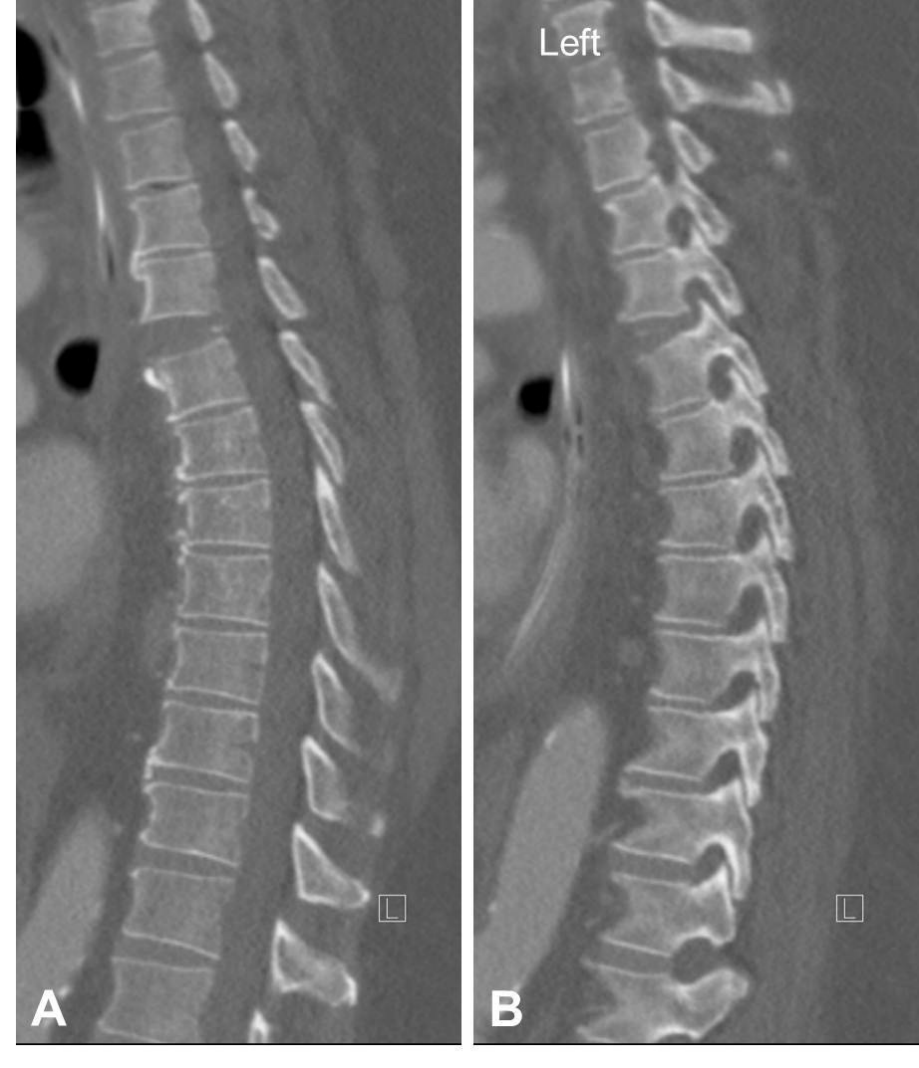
Sagittal Computerized Tomography (CT) of Injury A sagittal CT demonstrates extension type injury at T4/5 (A). A left parasagittal cut shows distraction of the facet joint at that level (B).
